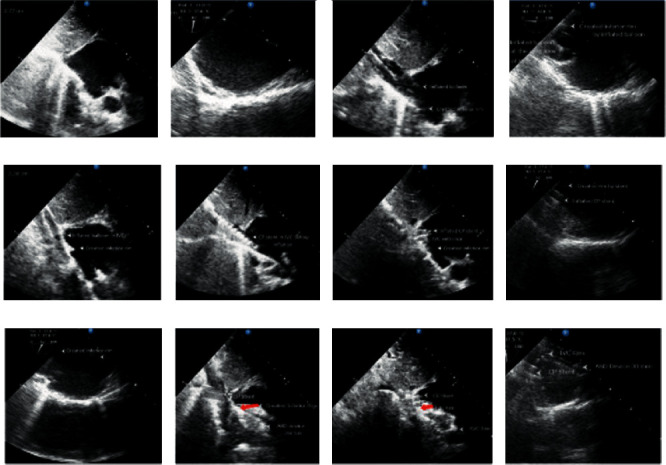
(a) Transthoracic echocardiorgraghy (TTE) imaging in subcostal view demonstrates a large secundum ASD with a totally absent inferior rim. (b) TEE imaging in long axis bicaval view demonstrates large secundum ASD. (c) TTE imaging in subcostal view demonstrates the inflated Z-med balloon with the created inferior rim (arrows). (d) TEE in modified oblique plane demonstrates the inflated balloon at the entrance of IVC with created inferior rim. (e) TTE imaging in the subcostal bicaval view demonstrates the inflated balloon with a created inferior rim. (f) TTE imaging in subcostal bicaval view shows the mounted uncovered CP stent at the entrance of the IVC before the inflation. (g) TTE imaging in subcostal bicaval view demonstrates the inflated uncovered CP stent at the entrance of the IVC with the created inferior rim. (h, i) TEE imaging in a modified long axis view (angle 67°) demonstrates the created inferior rim (upper arrow) by tight inflation of an uncovered CP stent at the entrance of the IVC (lower arrow). (j, k) TTE imaging in subcostal long axis view demonstrates the atrial septal occluder in proper position capturing the created inferior rim (red arrow). (l) TEE imaging in long axis bicaval view demonstrates the proper position of the atrial septal occluder capturing the new inferior rim (upper arrow) which has been created by inflation of uncovered CP stent (lower arrow) at the entrance of IVC.
